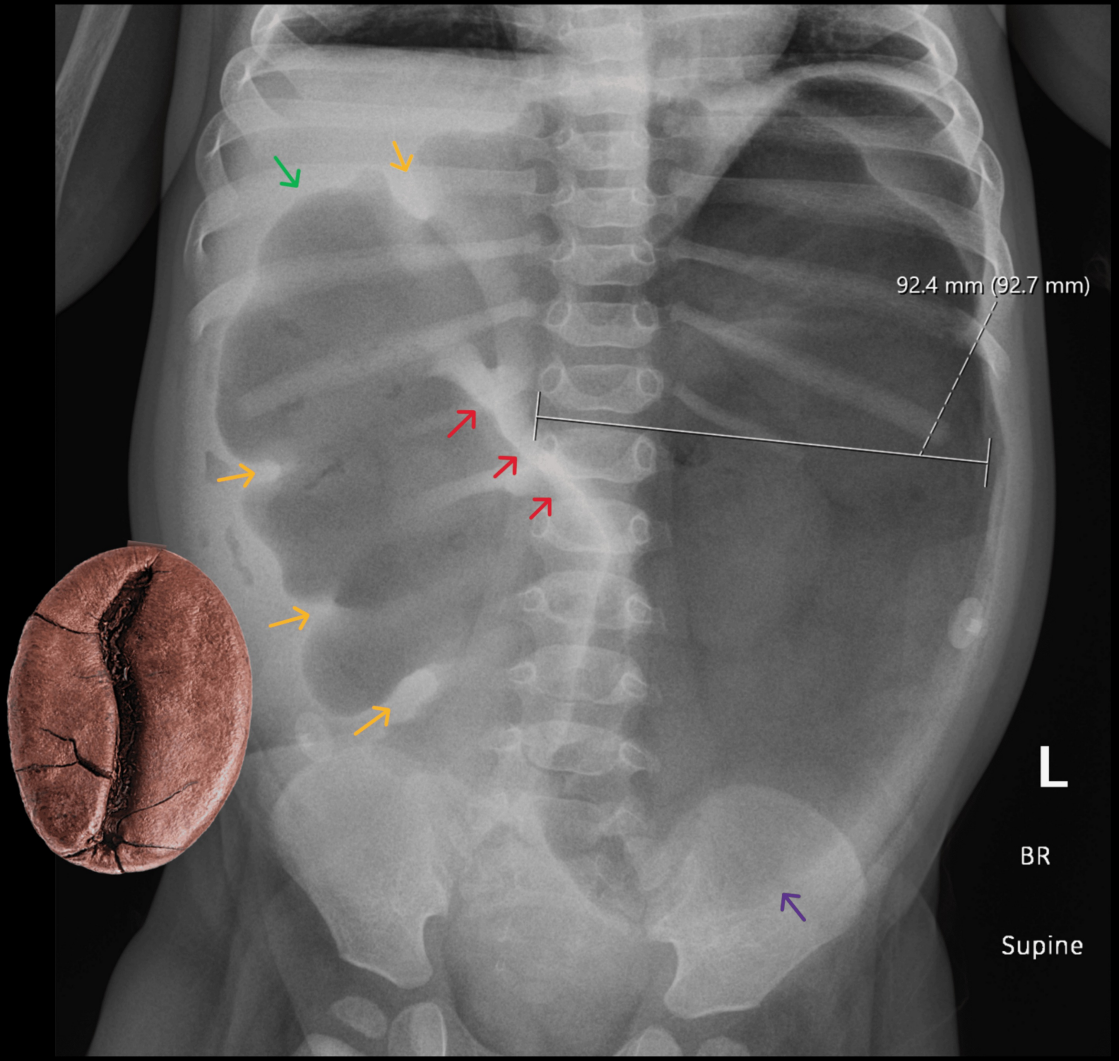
Abdominal radiograph (AP view) demonstrates marked gaseous distension of the large bowel, measuring up to 9.2 cm (on-image calipers). The dilated bowel is identified as the proximal segment of the transverse colon, as indicated by characteristic colonic haustral folds (yellow arrows) and the typical course of the bowel from the hepatic flexure (green arrow). An obliquely oriented “white stripe” sign is seen (red arrows), representing apposed walls of a gas-filled colonic loop resulting from a bowel twist. Overall, the distended proximal transverse colon segment demonstrates a coffee-bean configuration, with its apex directed toward the left iliac fossa (purple arrow), highly concerning for volvulus. Note that this orientation should not be confused with the classic “coffee-bean (omega)” sign seen typically in cases of sigmoid volvulus, in which the apex usually points to the right upper quadrant. AP = anteroposterior
